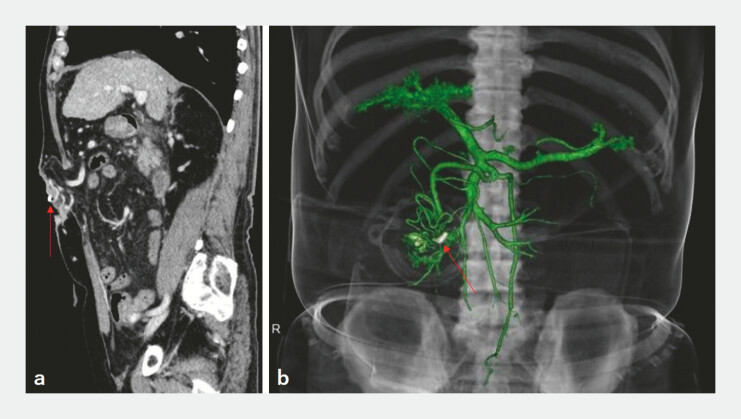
Contrast-enhanced computed tomography findings.
